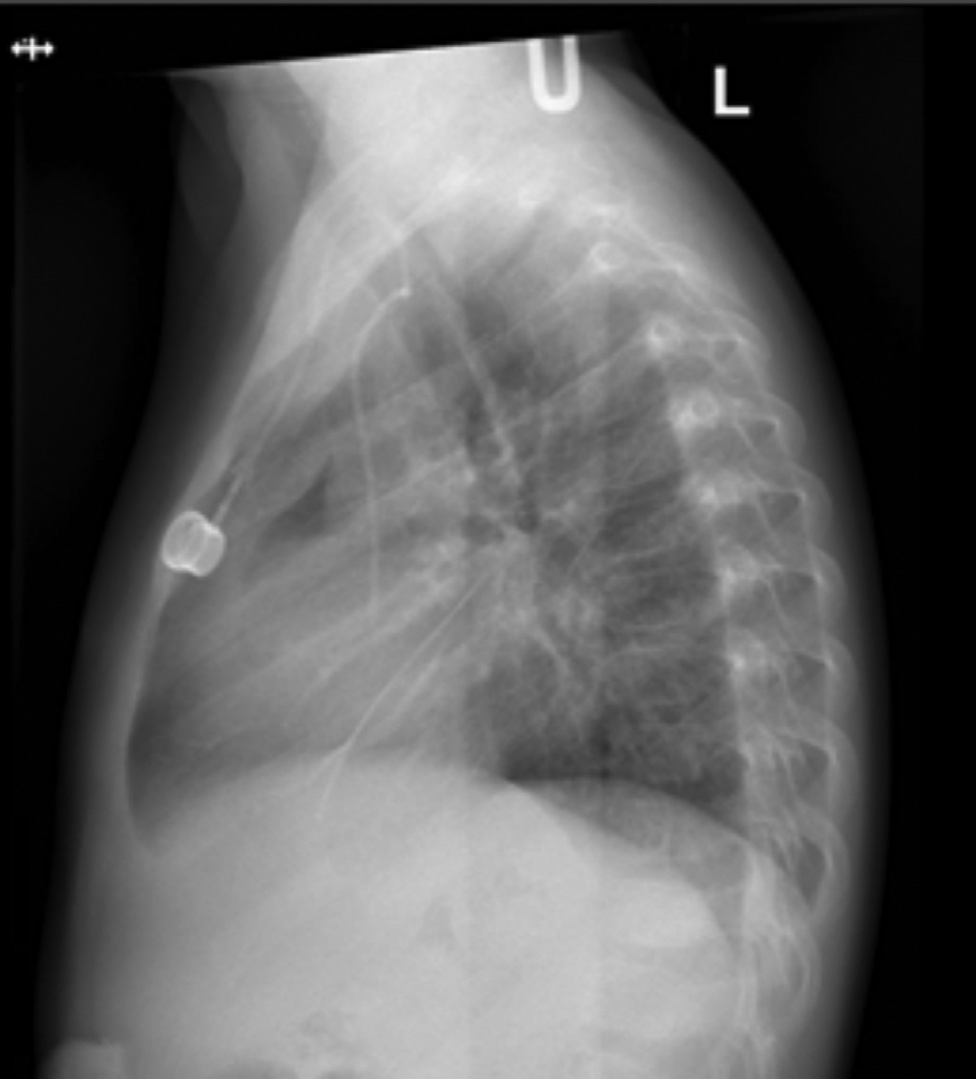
Lateral view of chest X-ray on day 0 postesophageal dilatation demonstrating lucency in the middle mediastinum, which is suggestive of esophageal perforation.
